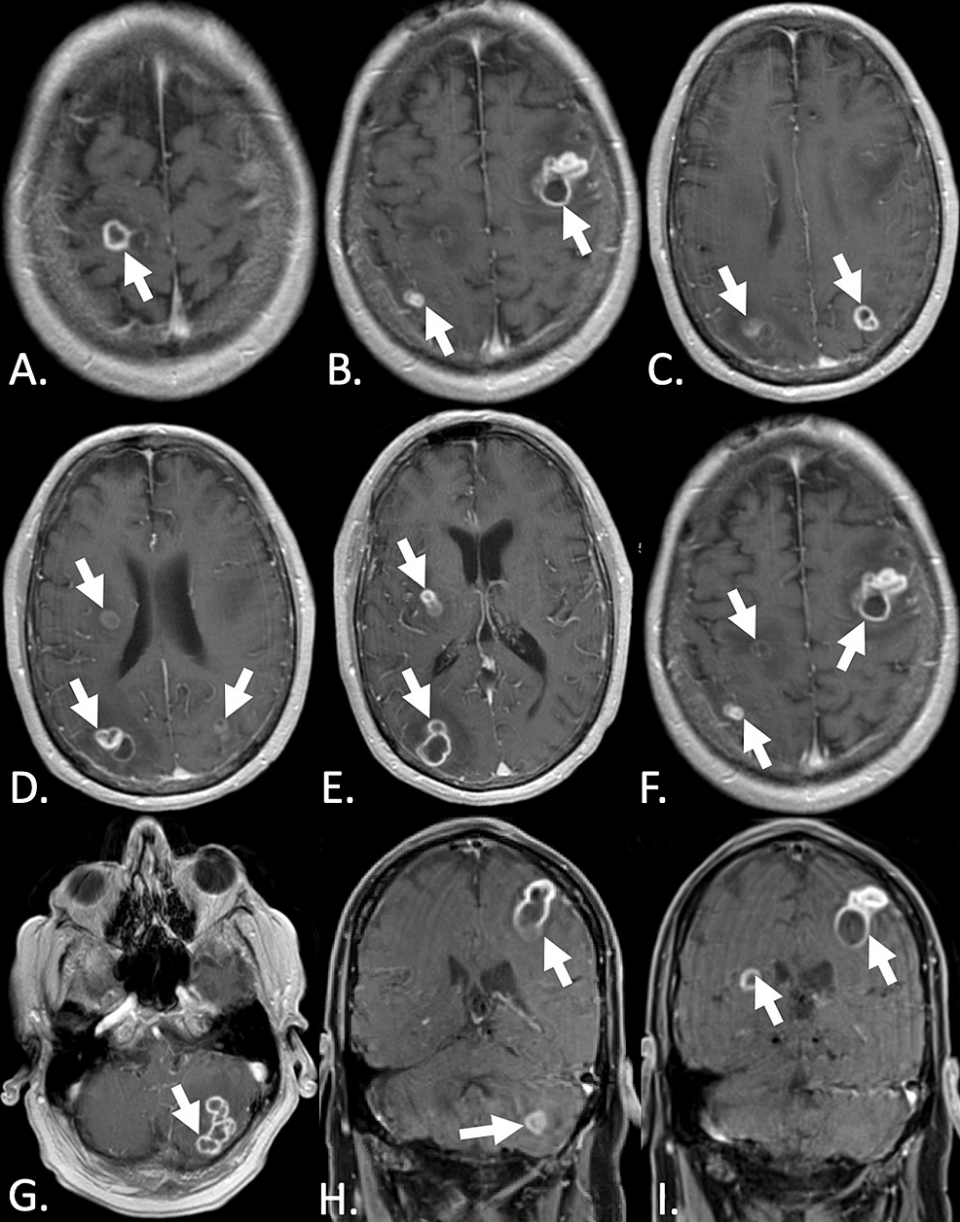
MRI of the brain: horizontal (A-G) and coronal (H&I) MRI sections with arrows indicating multiple ring-enhancing lesions
